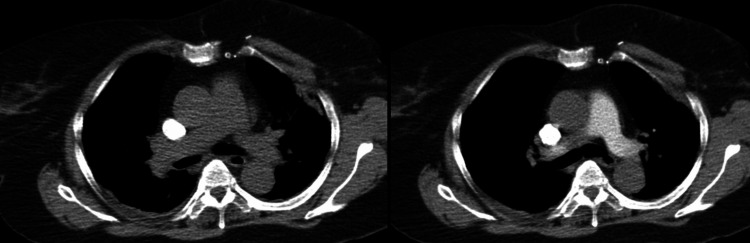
CT pulmonary angiogram. Multi-slice images from a CT pulmonary angiogram. The study was negative for pulmonary embolism.
